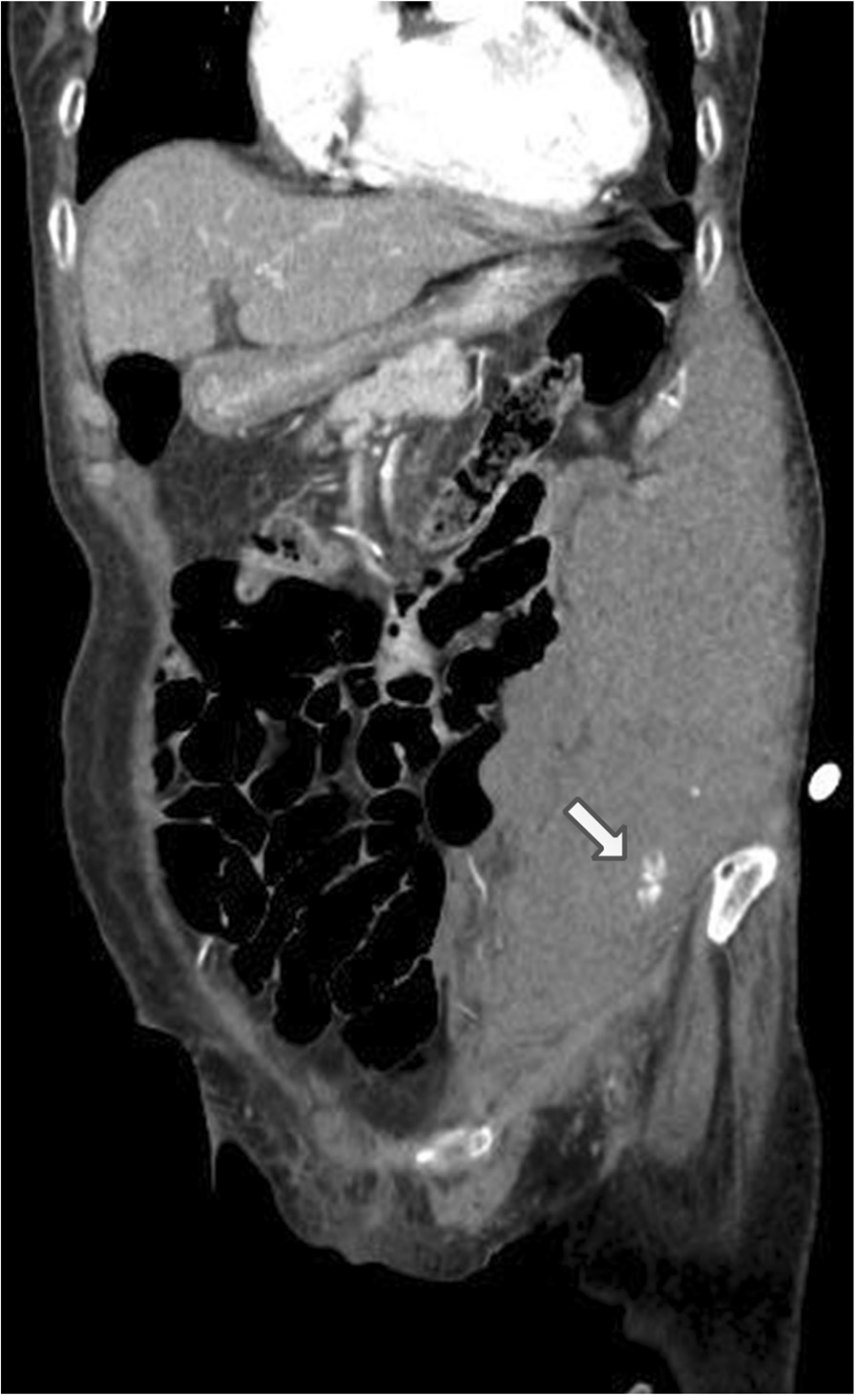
Abdominal CT with contrast showing a large hematoma and contrast extravasation (arrow) in abdomen.
